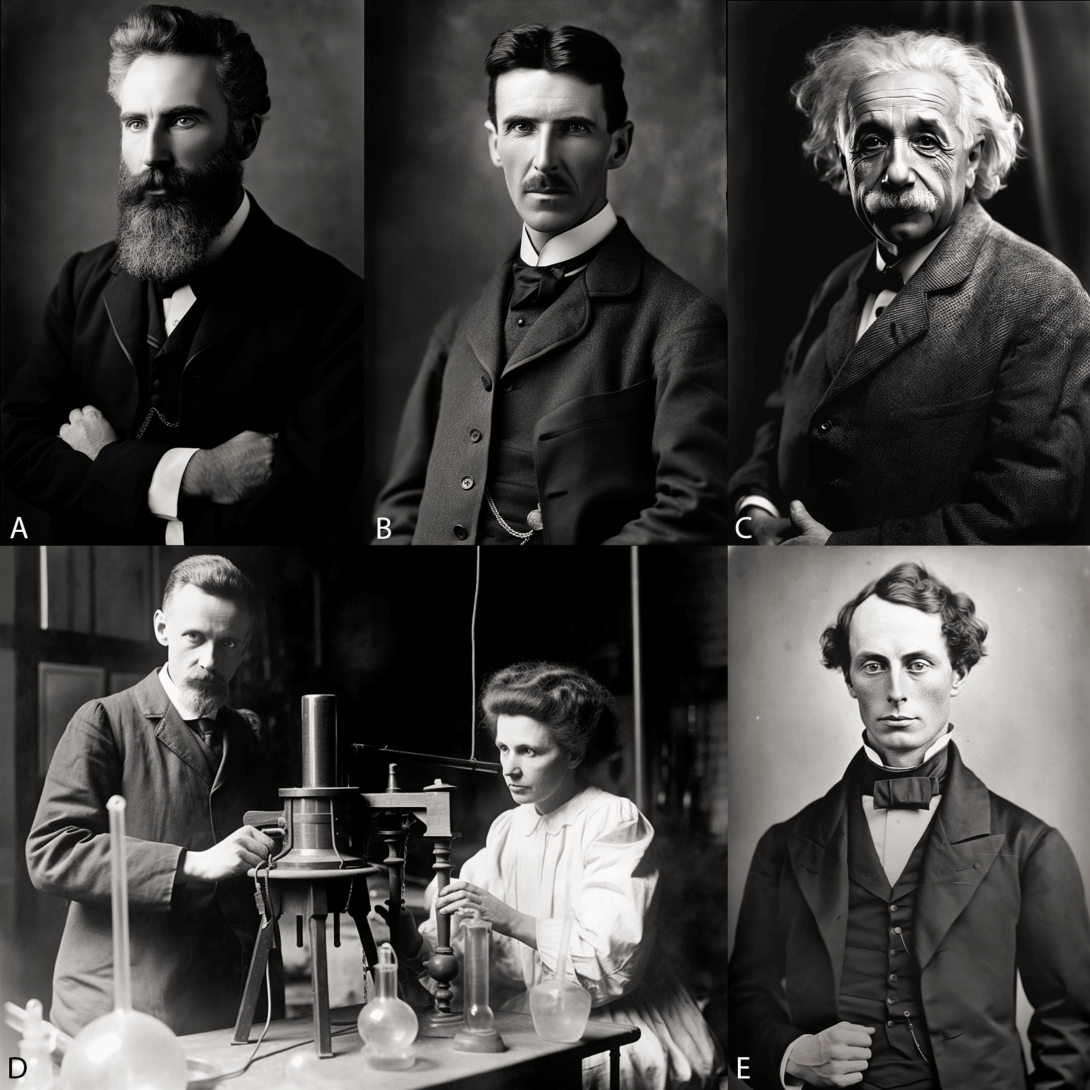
Visionary physicists in radiology AI-generated images using Midjourney v5.2 for generating photorealistic portraits of A. Wilhelm Conrad Roentgen, B. Nikola Tesla, C. Albert Einstein, D. Pierre and Marie Curie, and E. Christian Doppler (top left to bottom right). A combination of image-to-image and text-to-image capabilities were deployed using the ‘/imagine’ command, incorporating ‘--style raw’ and ‘--iw 2’ parameters. The ‘zoom out’ and ‘extend down’ capabilities were implemented to generate the lower half of the portraits showing the upper body. Of note, for Christian Doppler’s image, the ‘/blend’ command was implemented by blending a photograph and a sketch to generate more suitable results.
